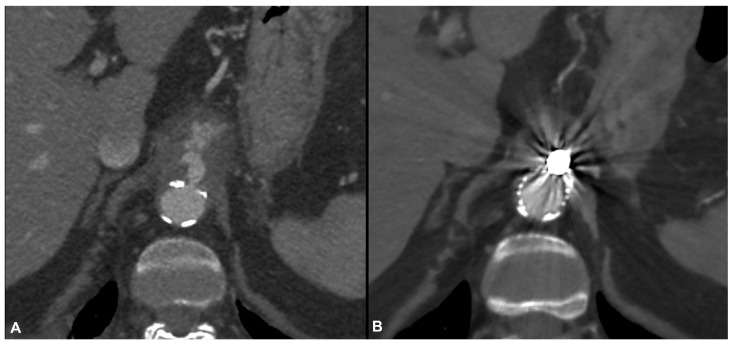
Pre-operative computed tomography angiography (CTA) of a contained ruptured supraceliac atheromatous plaque in a porcelain aorta (A) treated in emergency by TEVAR and occlusion of the celiac trunk by coils with good postoperative results as demonstrated by the postoperative CTA (B).
